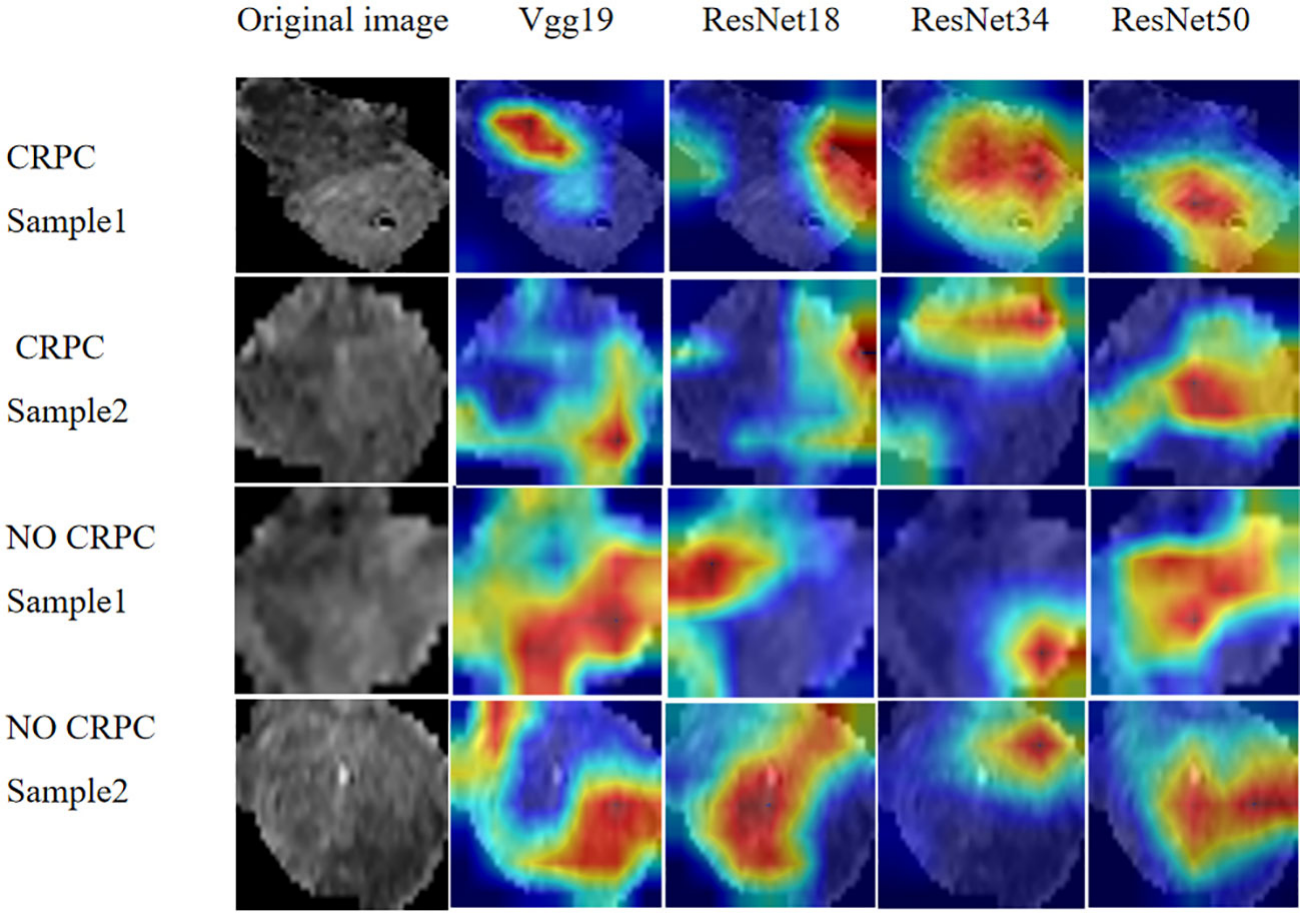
Regions of attention in prostate cancer MRI analysis with different DL models. MRI, magnetic resonance imaging.
